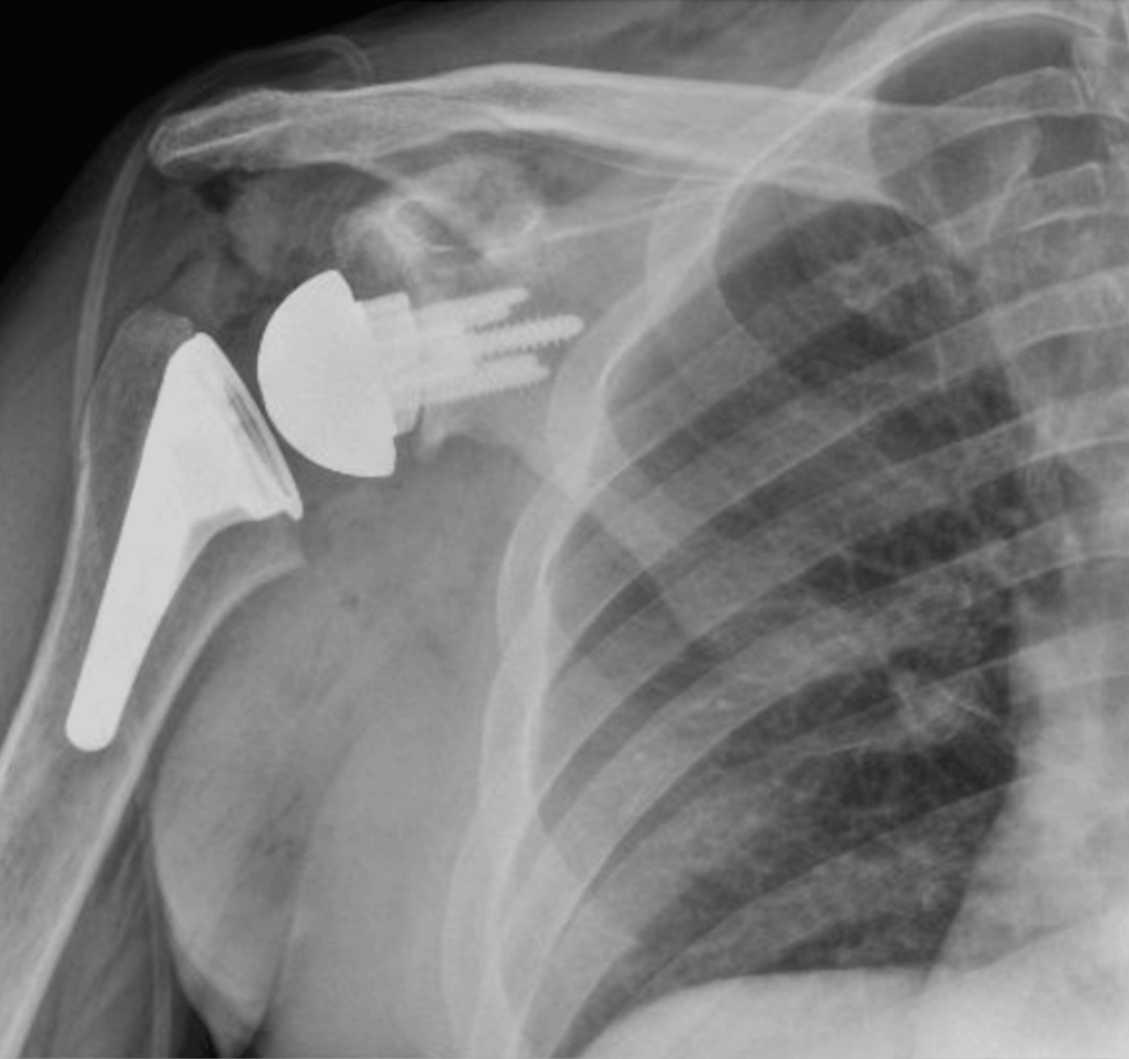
Postoperative X-rays of the right shoulder following reverse shoulder arthroplasty The image depicts an anteroposterior (AP) view of the right shoulder
